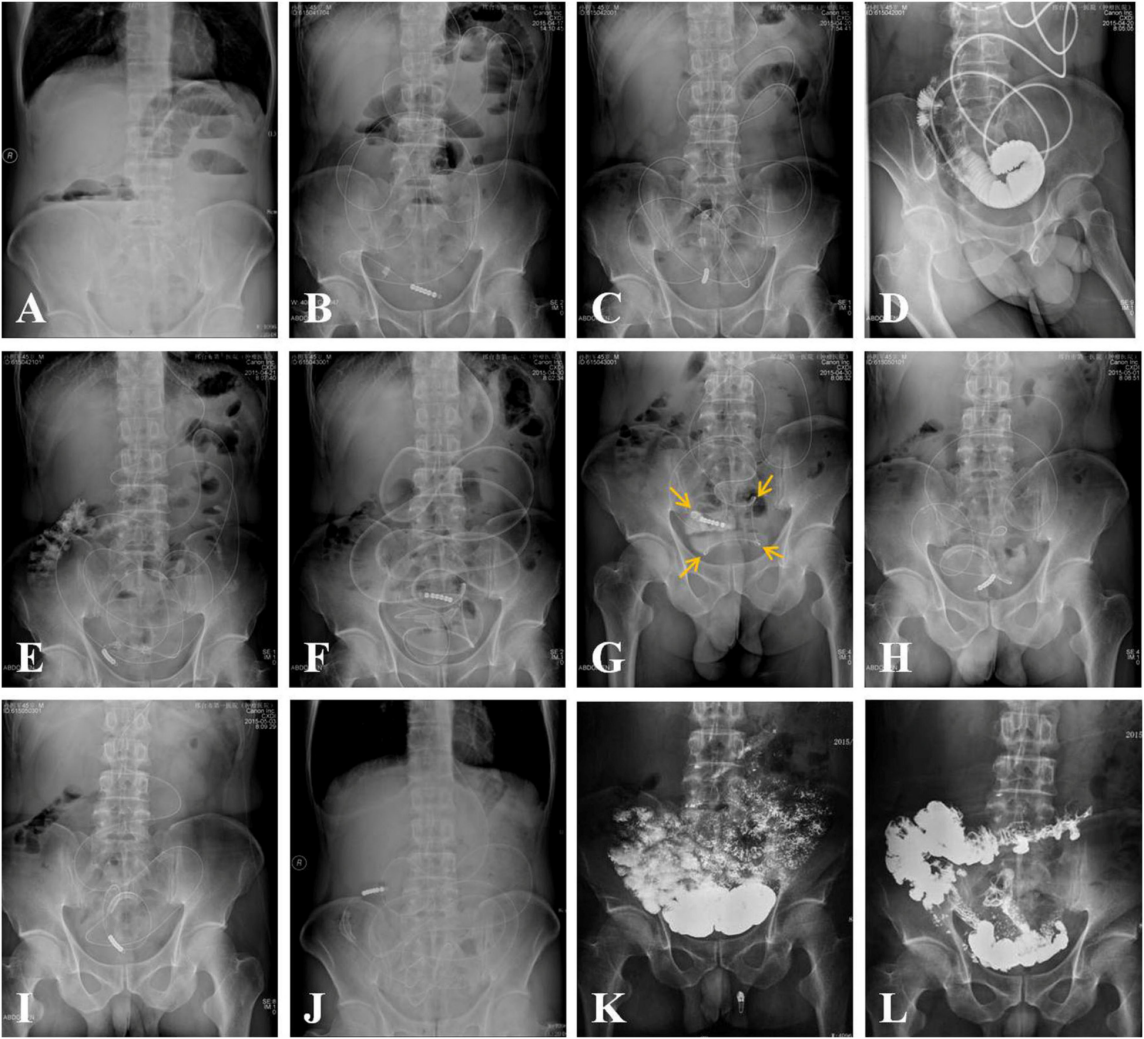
In a 45-year-old male patient, adhesive small bowel obstruction (ASBO) was observed two weeks following radical resection for appendicitis. (A) An erect abdominal plain film revealed evidence of small bowel obstruction. (B) A transnasal ileus tube was inserted into the small bowel under fluoroscopic guidance. (C) Three days post-placement, the advancement of the ileus tube's tip (indicated by an arrow) ceased. (D) An angiogram utilizing 76% meglumine diatrizoate demonstrated a complete obstruction of the small bowel. (E) After 24 h, the meglumine diatrizoate reached the right colon, indicating a transition from complete to incomplete small bowel obstruction. (F) Fourteen days after the ileus tube was placed, its tip reached the distal end of the small bowel. (G) Upon retracting the ileus tube, four obstructive sites (indicated by arrows) were identified. (H-J) Erect abdominal plain films taken 15, 20, and 24 days post-placement showed a gradual loosening of the adhesive obstructions. As the ileus tube was manipulated back and forth, the curvature of the bowel increased, the tortuosity decreased, and the fixed curvatures diminished. Ultimately, the ileus tube was expelled through the anus. (K,L) Gastroenterography demonstrates unobstruction of small bowel 6 months after the procedures.
